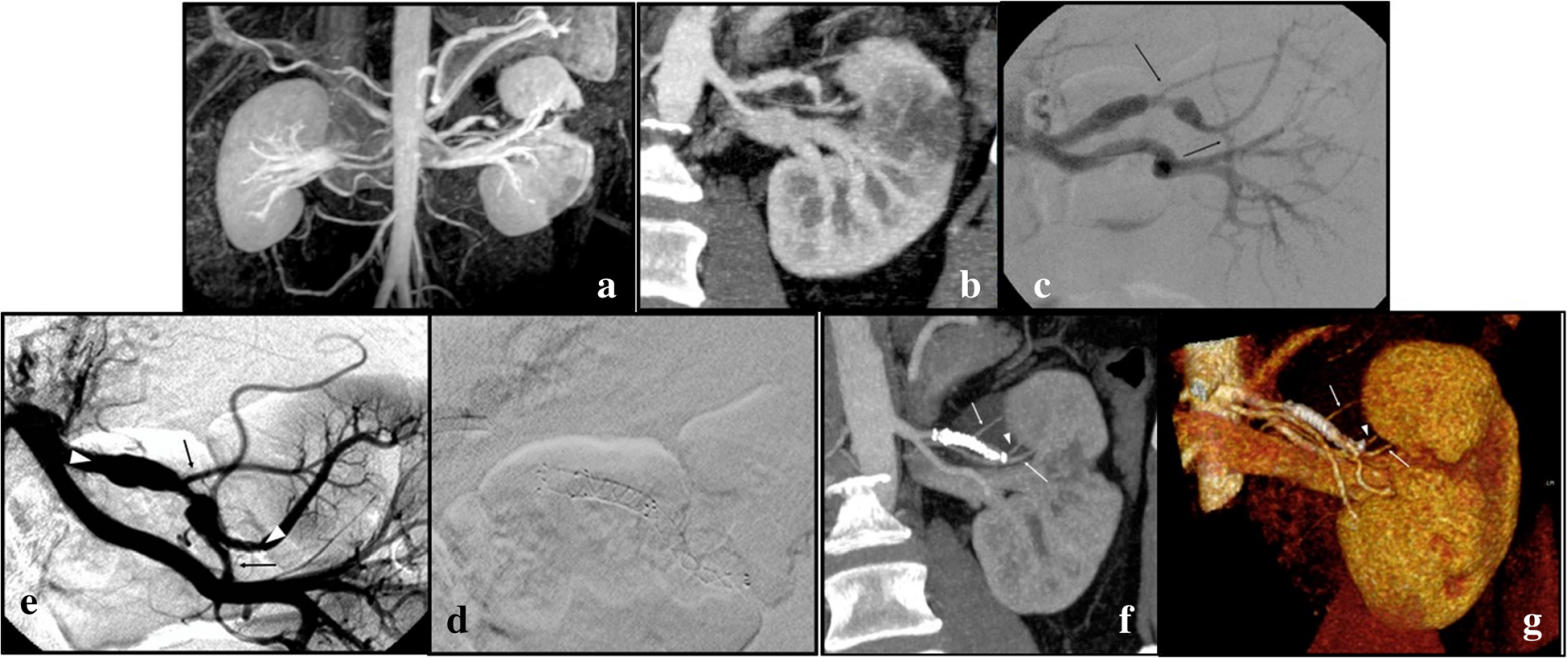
a-g Coronal CTA (a) and 3D MRA imaging (b) at baseline, showing a bilobate VAP of the upper branch of the left renal artery and an ipsilateral hypodense-hypointense wedge-shaped parenchymal area of renal infarction. Selective renal artery DSA (c–e) before and after the deployment of two Fred 4/18/12 mm and 3.5/31/24 mm flow diverter stents, which are placed across two branching vessels (arrows), arising from the pseudoaneurysmatic artery (arrowheads in (e) show distal and proximal landing zone of the stents). Coronal CTA and VR imaging (f-g) at the 12-months follow-up show complete exclusion of the VAP, the parent vessel distally to the FDS (arrowhead) is patent as well as the stented sidebranches (arrows)
